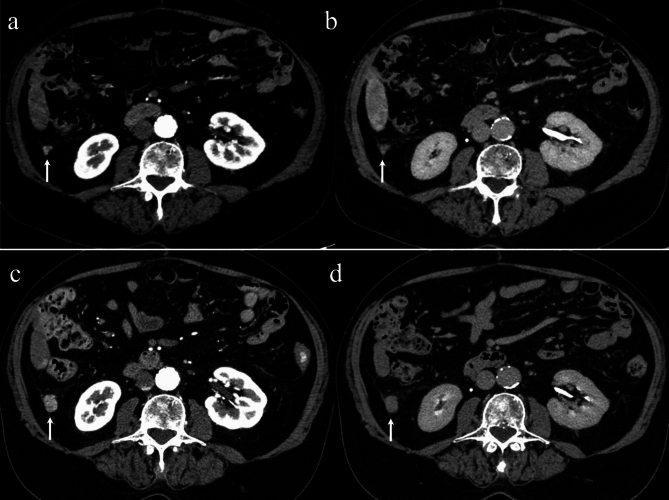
Contrast-enhanced computed tomography (CT) imaging shows a 5 mm peritoneal nodular lesion along the right lateroconal fascia in both the arterial (arrow in a) and portal-venous (arrow in b) phases. The nodule may represent an extrahepatic HCC metastasis possibly secondary to tumor seeding following a prior radiofrequency ablation (RFA). Contrast-enhanced CT performed 3 months after the transarterial chemoembolization of the two intrahepatic nodules demonstrates a notable growth of the extrahepatic deposition (10 mm vs. 5 mm) (arrows in c and d)
